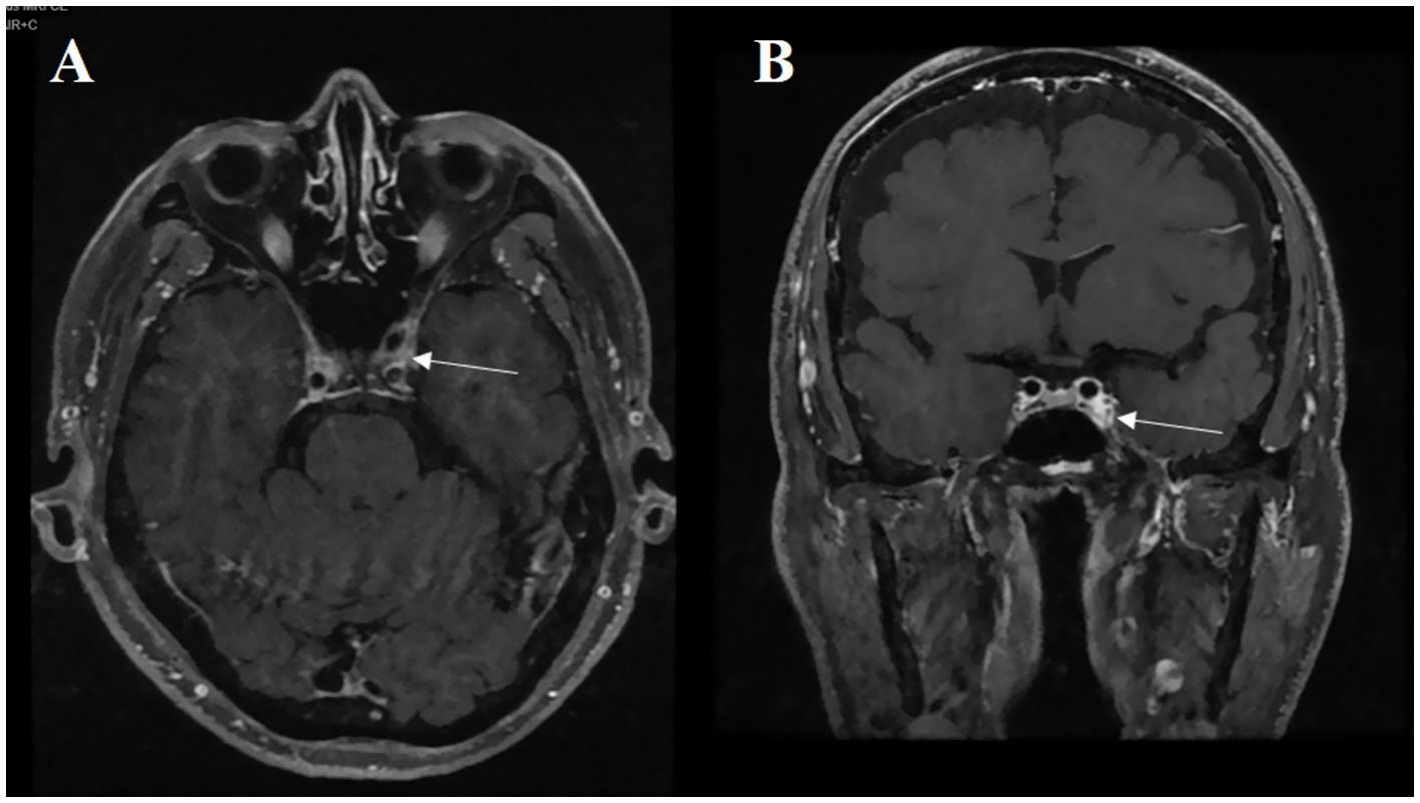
A 74-year-old male patient presenting with vertical diplopia. (A) The abnormal asymmetric high intensity was found in the cavernous sinus on the enhanced axial T1-weighted image (white arrow). (B) On the enhanced coronal T1-weighted image, we can observe the same abnormal phenomenon (white arrow).
